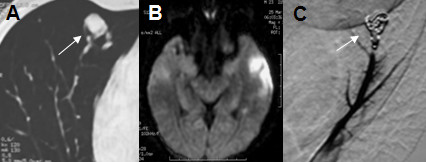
Radiological features of patient 1. A: Contrast-enhanced thoracic CT scan shows PAVMs (arrow); B: Brain MRI diffusion hyperintensity in the left temporal cortical region; C: Pulmonary angiography showing PAVMs after embolization (arrow).
